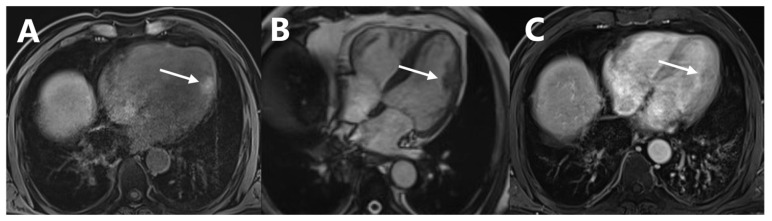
Dynamic myocardial perfusion MRI ((A) T1WI, (B) T2WI, (C) delayed scan of enhanced T1WI) also detected the lesion in the left ventricular lateral wall with a short T1 signal and a long T2 signal intensity with delayed enhancement (Figure 2, arrows). Subsequent gene testing revealed an insertion mutation in exon 11 of the KIT gene and no BRAF mutations were detected. Considering the advanced stage of malignant melanoma, he was treated with imatinib and received radiotherapy of the largest mass in the right lung. However, the treatment of imatinib was discontinued 6 weeks later because of a drug reaction with eosinophilia and systemic symptoms (DRESS). The patient experienced rapid progression of the tumor 4 months thereafter and died of the disease.
